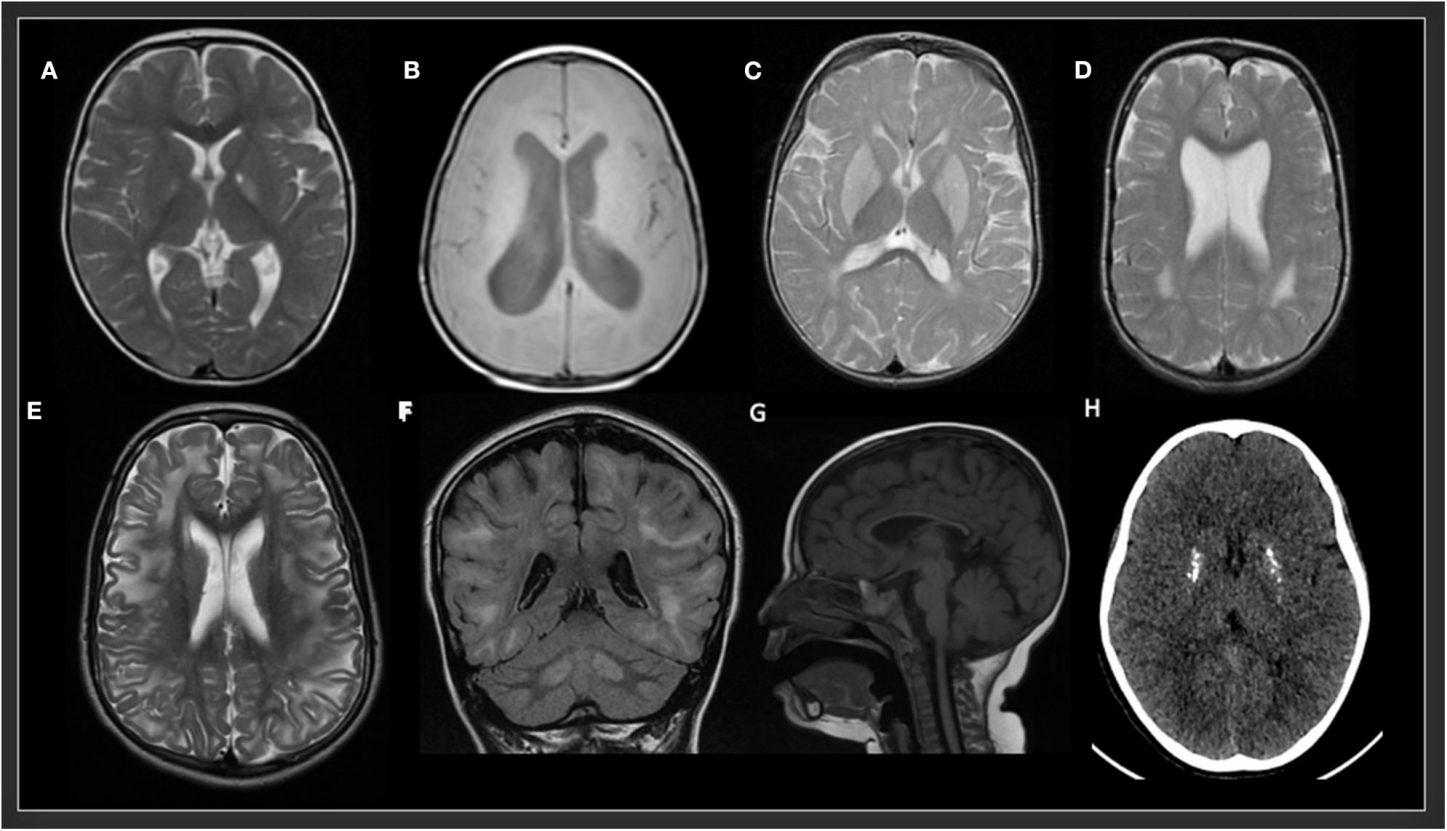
Radiological patterns in selected inborn errors of metabolism. (A) Mitochondrial short-chain enoyl-CoA hydratase 1 deficiency (*ECHS1*). MRI (T2W) showing bilateral symmetric signal hyperintensity in globus pallidus and a small cavitation in the left globus pallidus. (B) Type 3 Gaucher disease (*GBA*). MRI (T1W) showing decrease volume of white matter and hydrocephalus. (C) Glutaric aciduria type 1 (*GCDH*). MRI (T2 FSE) showing bilateral symmetric signal hyperintensity in putamen and globus pallidus and posterior periventricular white matter abnormalities. (D) Rhizomelic chondrodysplasia punctata, type 1 (*PEX7*). MRI (T2) showing bilateral symmetric posterior periventricular white matter hyperintensity and ventriculomegaly. (E,F) L-2-hydroxyglutaric aciduria (*L2HGA*). MRI (T2 and FLAIR) showing bilateral diffuse cerebral white matter and dentate nuclei hyperintensities. (G) Methylmalonic aciduria and homocystinuria, cblC type (*MMACHC*). MRI (T1) showing a very thin corpus callosum. (H) Aicardi-Goutières syndrome 2 (*RNASEH2B*). CT showing multiple calcifications in basal ganglia.
